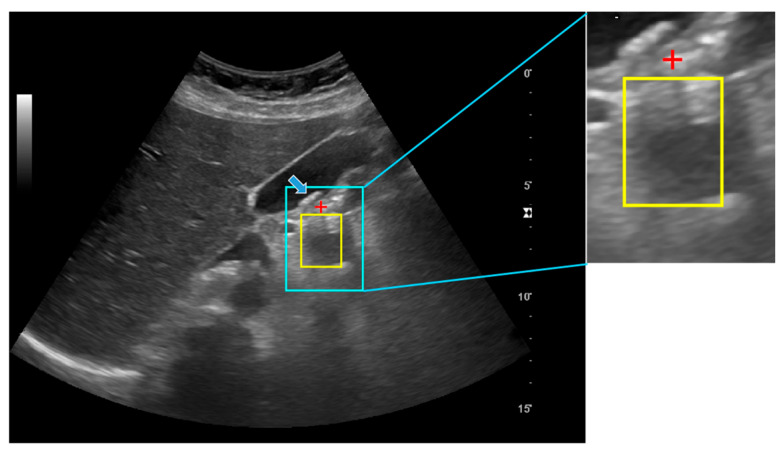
Fail case of automatic stone localization and posterior acoustic shadow detection using the proposed method and its enlarged image in gallbladder ultrasound image.
